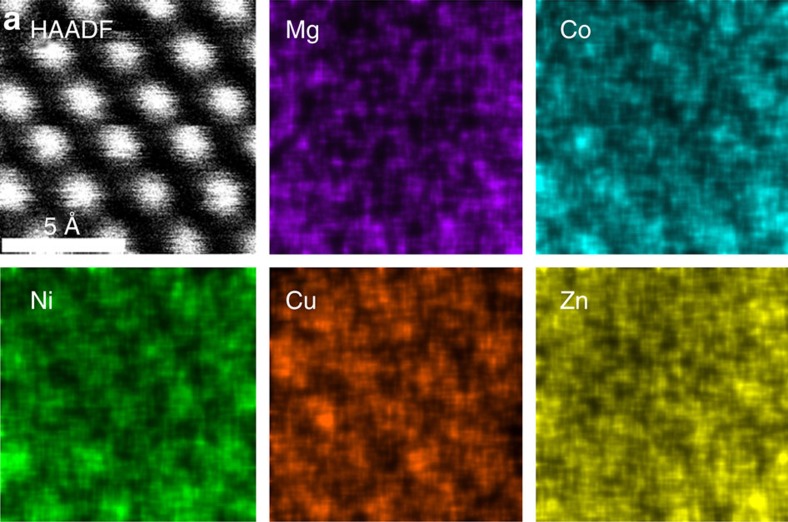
STEM–EDS analysis of E1. (a) HAADF image. Panels labelled as Zn, Ni, Cu, Mg and Co are intensity maps for the respective characteristic X-rays. The individual EDS maps show uniform spatial distributions for each element and are atomically resolved.
